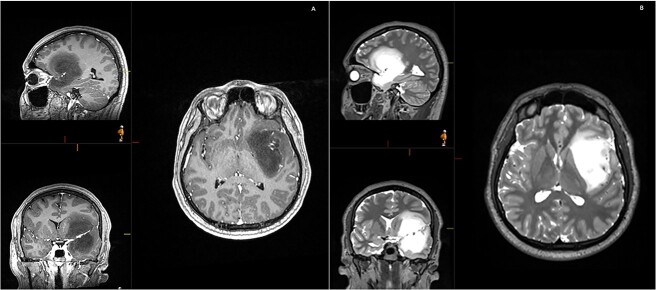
Initial MRI demonstrating a non-contrast enhancing left frontotemporal insular lesion. (A) T1-weighted post contrast; (B) T2-weighted.
